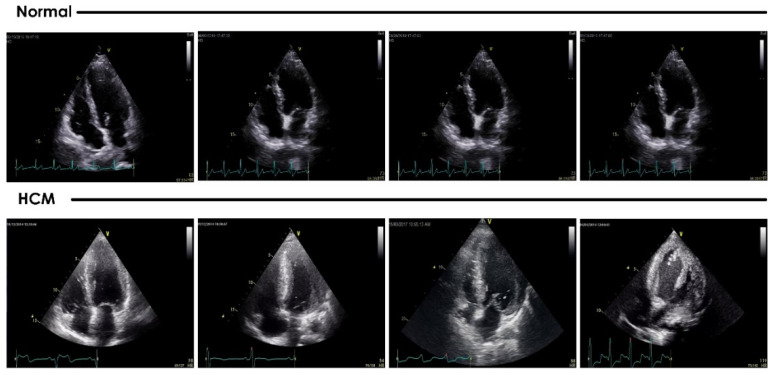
Example apical 4-chamber US images of normal versus hypertrophic cardiomyopathy participants.
